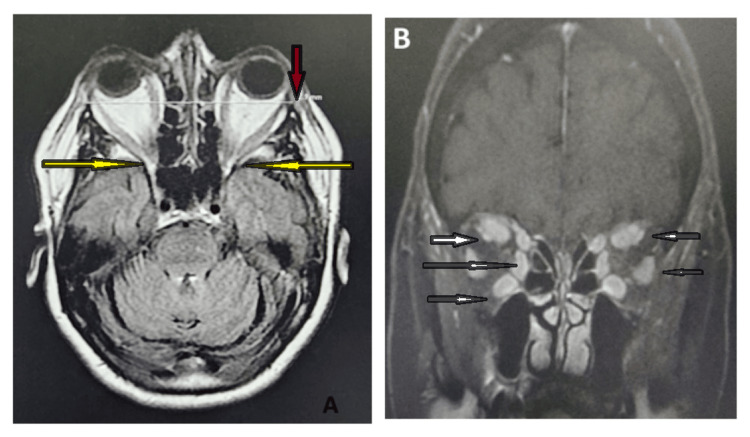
Orbital MRI axial and coronal view showed bilateral proptosis (brown arrow), an enlargement of all the extraocular muscles with an orbital fat hypertrophy (white arrows) and a stretching of the optic nerve on both sides (yellow arrows) Image provided by the ENT, head and neck surgery department of Hassan II University Hospital of Fez, Morocco, taken during consultation
